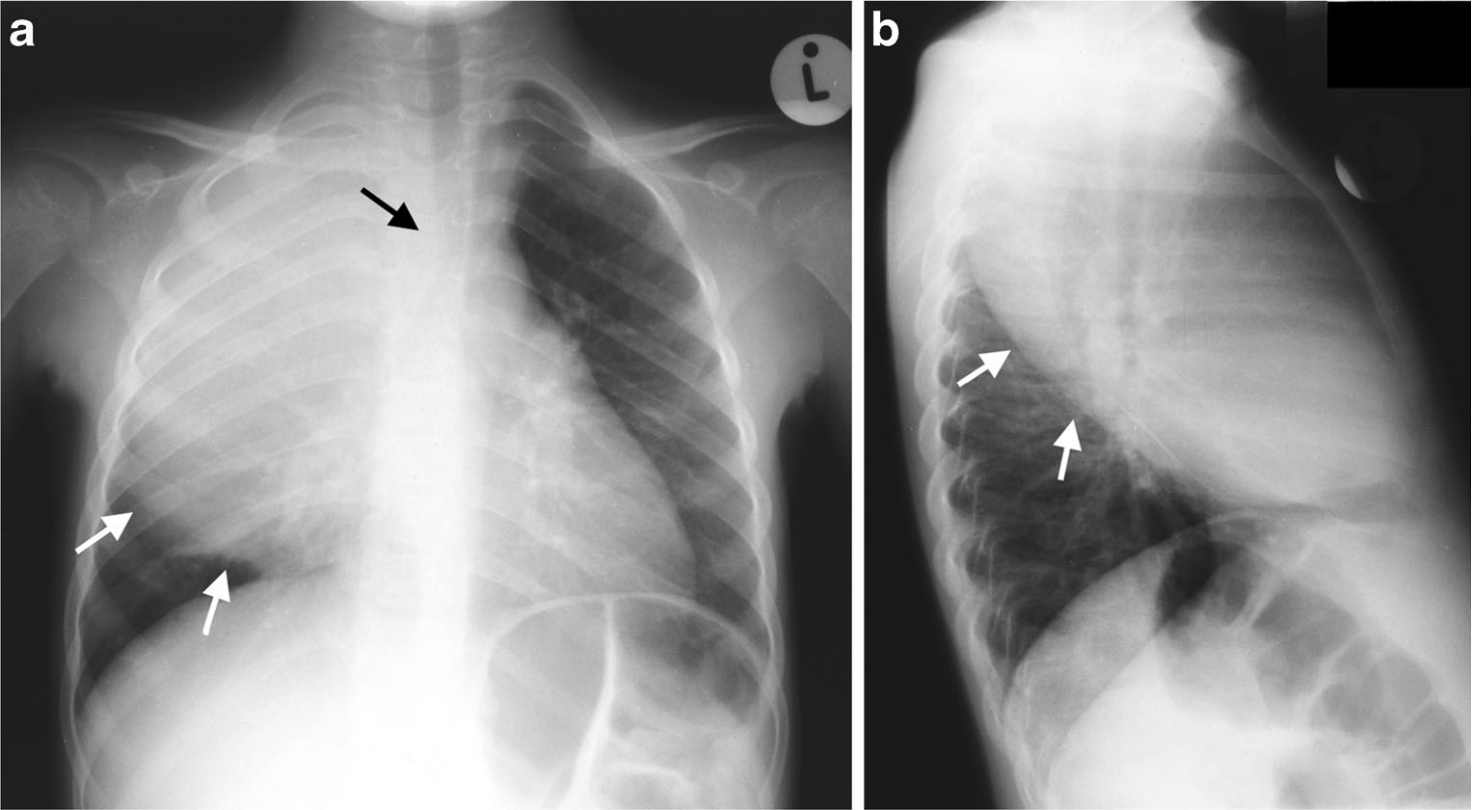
Chest radiographs in a 3-year-old boy who failed to respond to antibiotics and developed expansile pneumonia. Anteroposterior chest radiograph (a) and lateral chest radiograph (b) demonstrate dense opacity in the right upper lobe, a bulging inferior margin (*white arrows*) and mass effect on the mediastinal structures (*black arrow* in a). This was diagnosed as an expansile pneumonia, but the radiograph was unable to distinguish whether the underlying lung was congested or had undergone necrotic or suppurative change. The organism responsible was identified as *Klebsiella pneumoniae*
